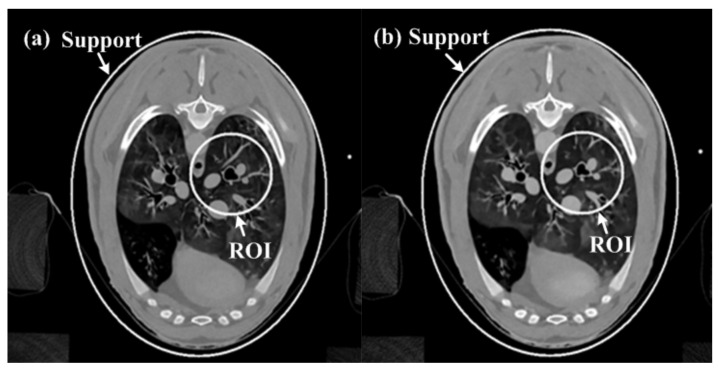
Reconstructed images from real CT projection data: (a) Normal dose; (b) low dose. The display window is [−700 800]HU.
